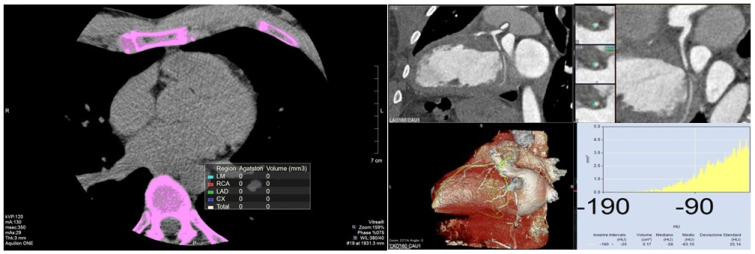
A 45-year-old male with a history of hypertension and hyperlipidemia presents for emergency evaluation. The patient is symptomatic for chest pain. A coronary computed tomography angiography was performed. The right image shows a coronary artery calcium score = 0 for all coronary arteries, indicating no detectable calcified plaque. In the left panel, the Multiplanar Reformation and Volume Rendering show a clear soft plaque on left circumflex artery. The elevated perivascular fat attenuation index indicates a vulnerable plaque. These findings suggest the absence of calcified plaque but the presence of a soft, potentially vulnerable plaque that may predispose the patient to future cardiovascular events.
